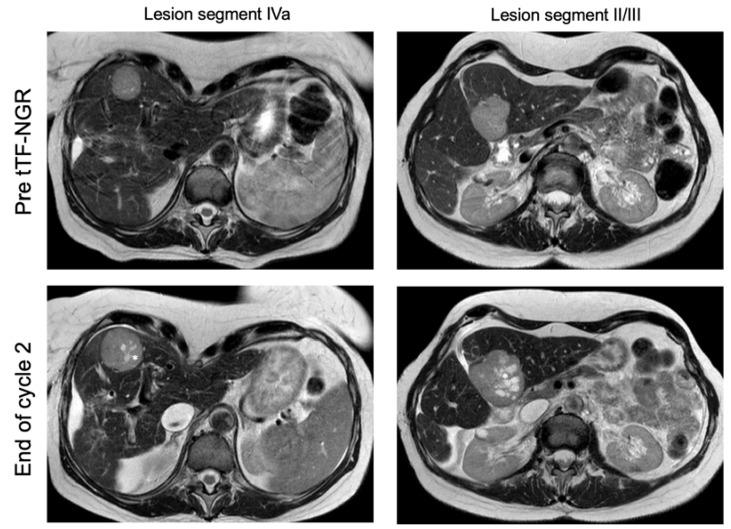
Morphological changes in hepatocellular carcinoma (HCC) (UPN 022) under tTF-NGR therapy: In the initial MRI scans, two prominent HCC lesions are visible in a transversal T2 weighted image. Before treatment with tTF-NGR, the HCC lesions are homogenously hyperintense as compared to the surrounding liver tissue (upper panels). After two cycles with tTF-NGR at 3.0 mg/m^2^ × 5, morphological changes with multiple hemorrhagic regions (*) have occurred inside the tumor lesions (lower panels), and at the same time, enlargement of tumor diameters can be seen.
